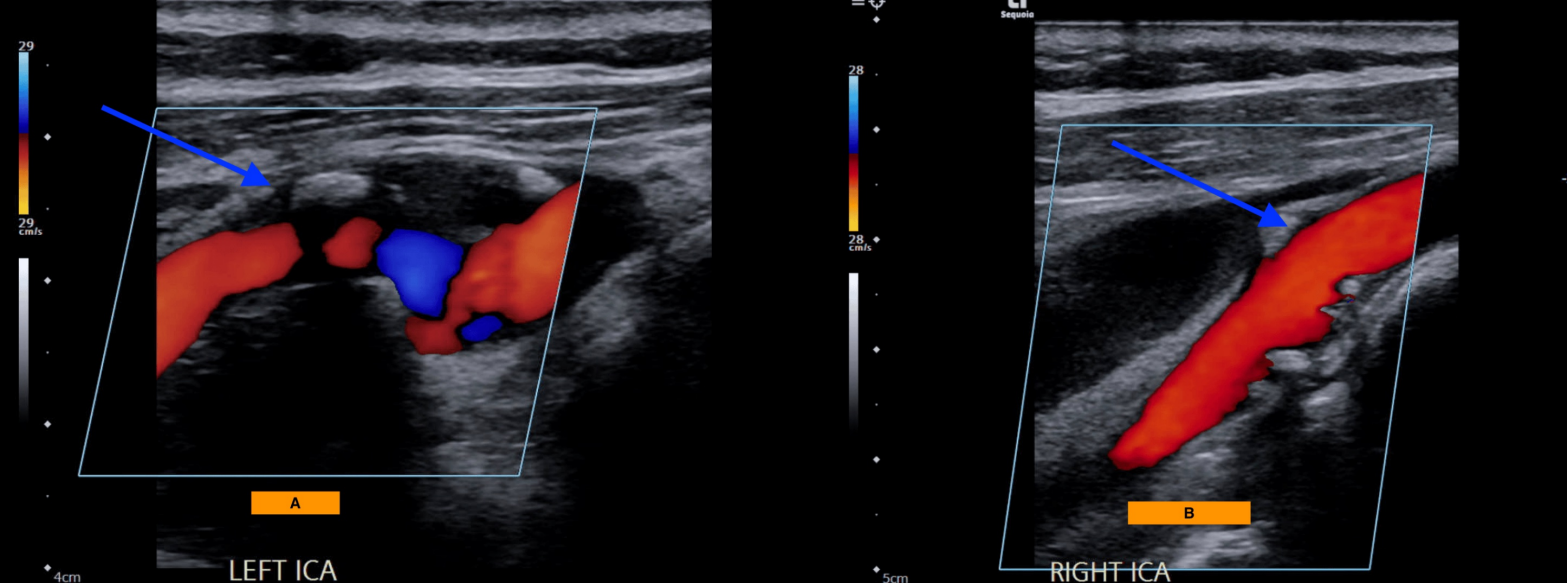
Case 1: Carotid artery ultrasound (A) <50% left ICA stenosis, heterogeneous plaque present (B) <50% right ICA stenosis, heterogeneous plaque present ICA: internal carotid artery
